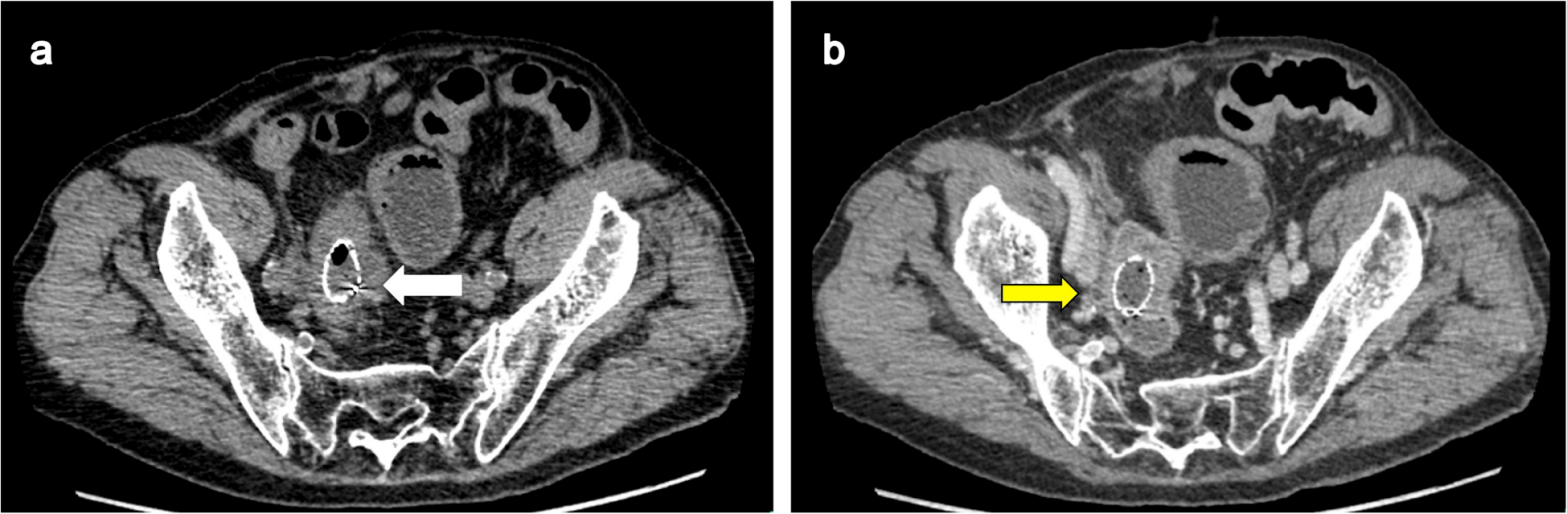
a Abdominal multi-detector computed tomography (CT) scan showing decompression of distended bowel and patency of self-expanding metallic stent (white arrow). b A contrast-enhanced CT image showing the existence of a tumor near the right ureter, with possible invasion (yellow arrow)
